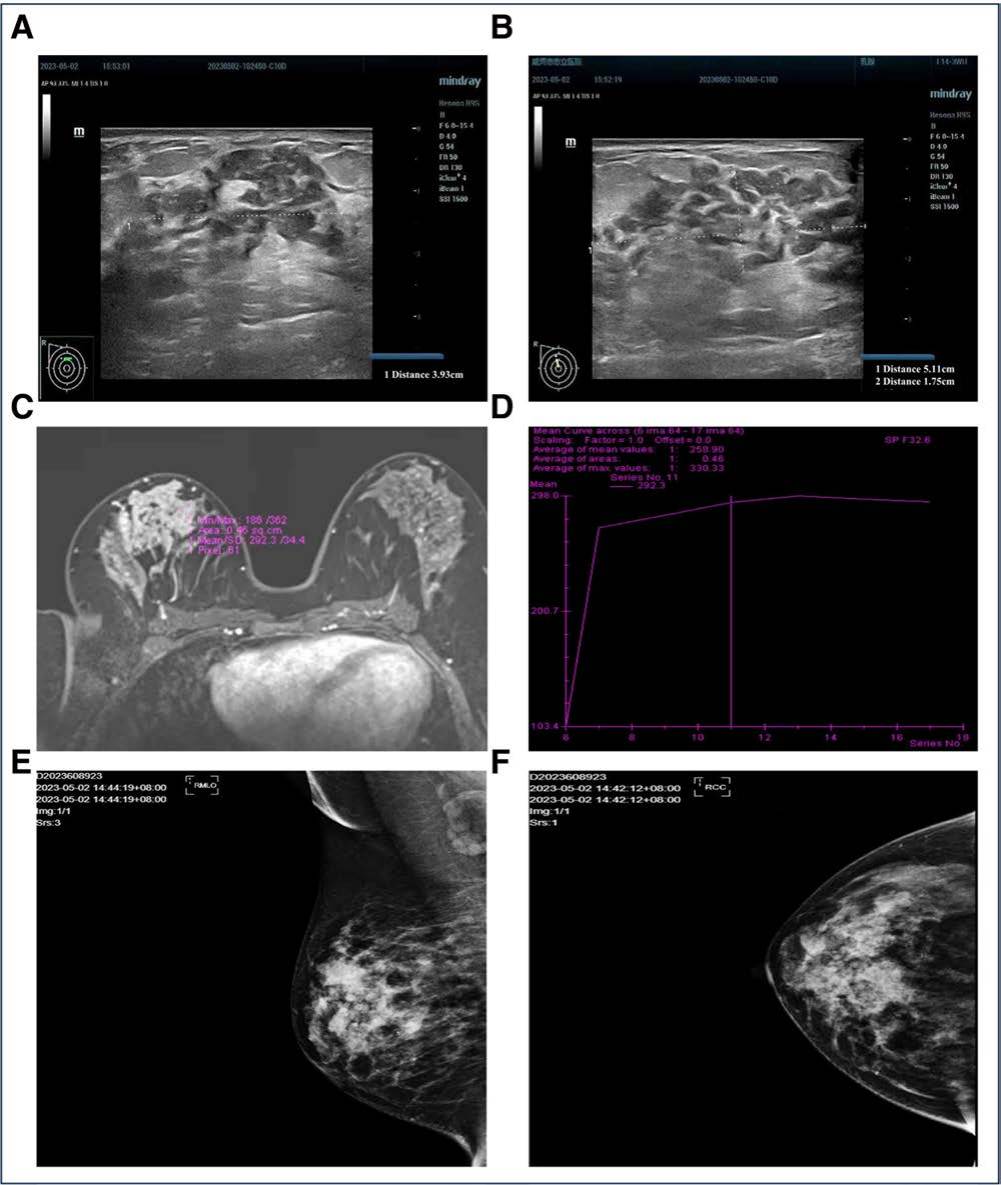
Imaging of breasts. (A and B) Color Doppler ultrasound of the right breast. (C) Radiographic mammography of bilateral breasts. Purple annotated area indicates the lesion in the right breast. (D) Breast magnetic resonance dynamic enhanced curve of purple annotated area in (C). (E) Medio-lateral oblique and (F) cranio-caudal molybdenum target X-ray image of right breast.
